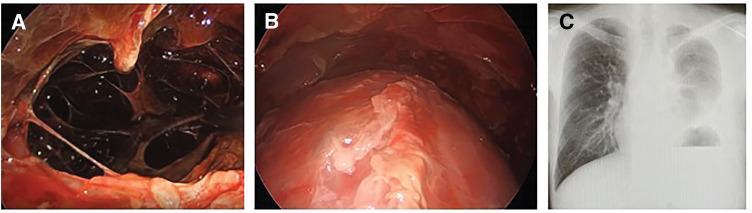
Intraoperative and postoperative findings. (A) Thoracoscopic findings showing a large amount of black pleural fluid and markedly thickened inflammatory pleura, consistent with empyema. (B) Thoracoscopic view after decortication showing satisfactory lung re-expansion before chest closure. (C) Chest radiograph at discharge showing good re-expansion of the left lung.
